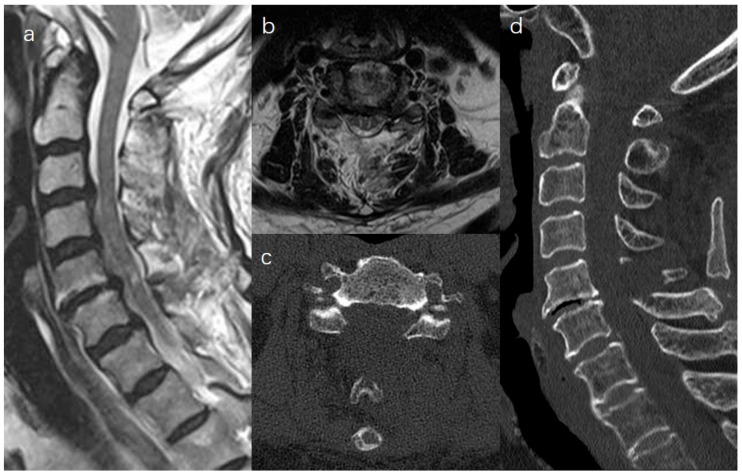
Postoperative MRI showed hypertrophied LF was removed (a,b). A CT scan revealed that laminectomy was performed, while PTB and cervical lordosis were preserved (c,d).
